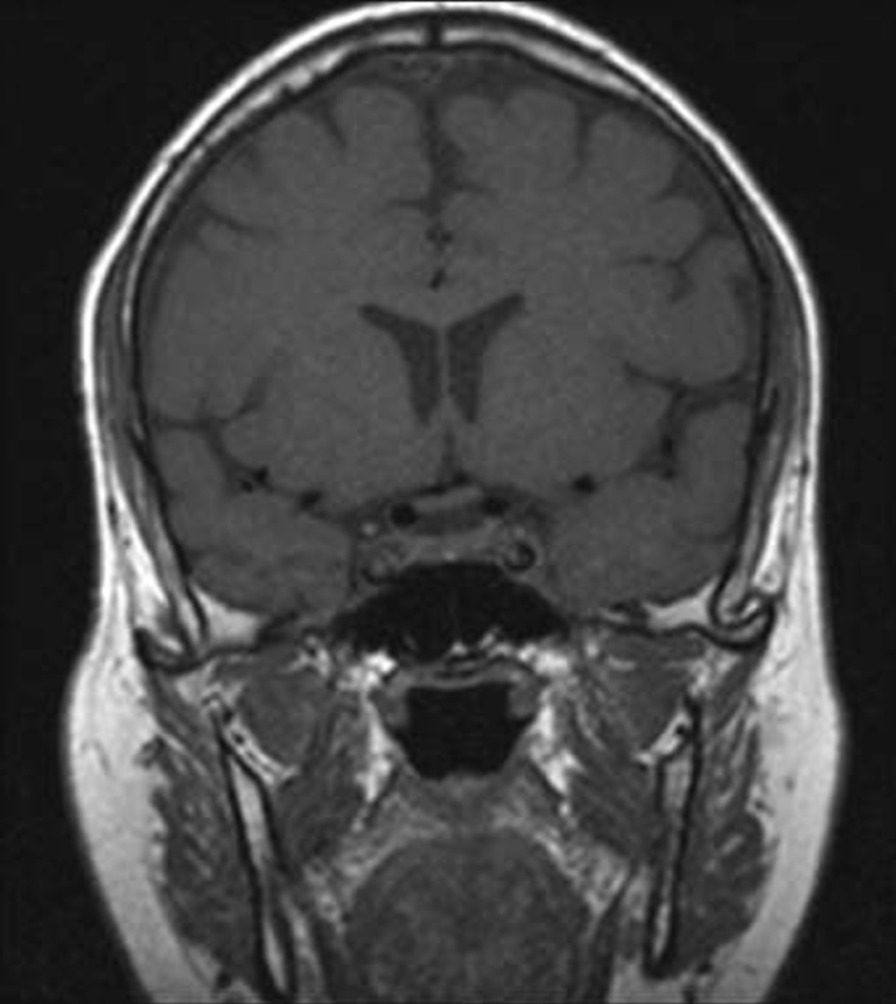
Post COVID-19 MRI findings. The image shows improvement of the pituitary microadenoma. The changes included the disappearance of the hypointense lesion and hyperintense enhancement on contrast observed on previous MRI. No macroadenoma or microadenoma is observed
